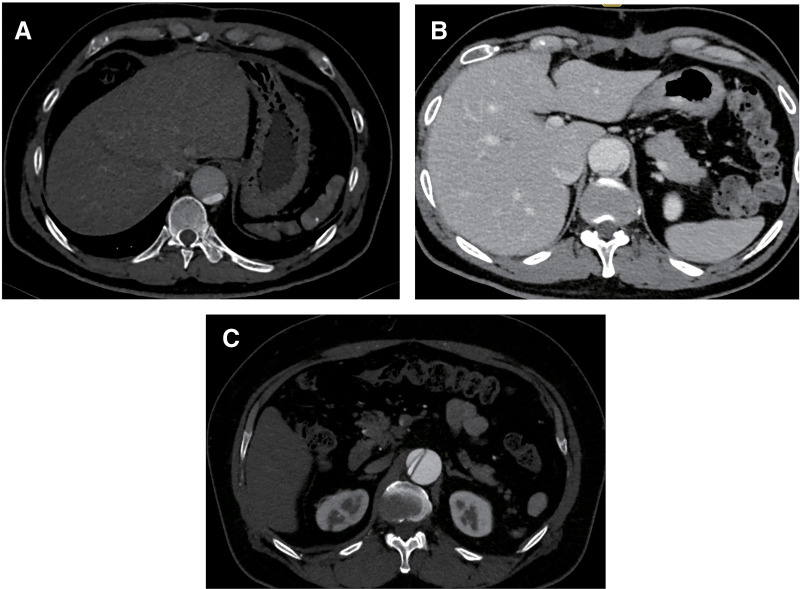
(A–C) Postoperative computed tomography images of 3 patients showing persistent collapsed true lumen.
